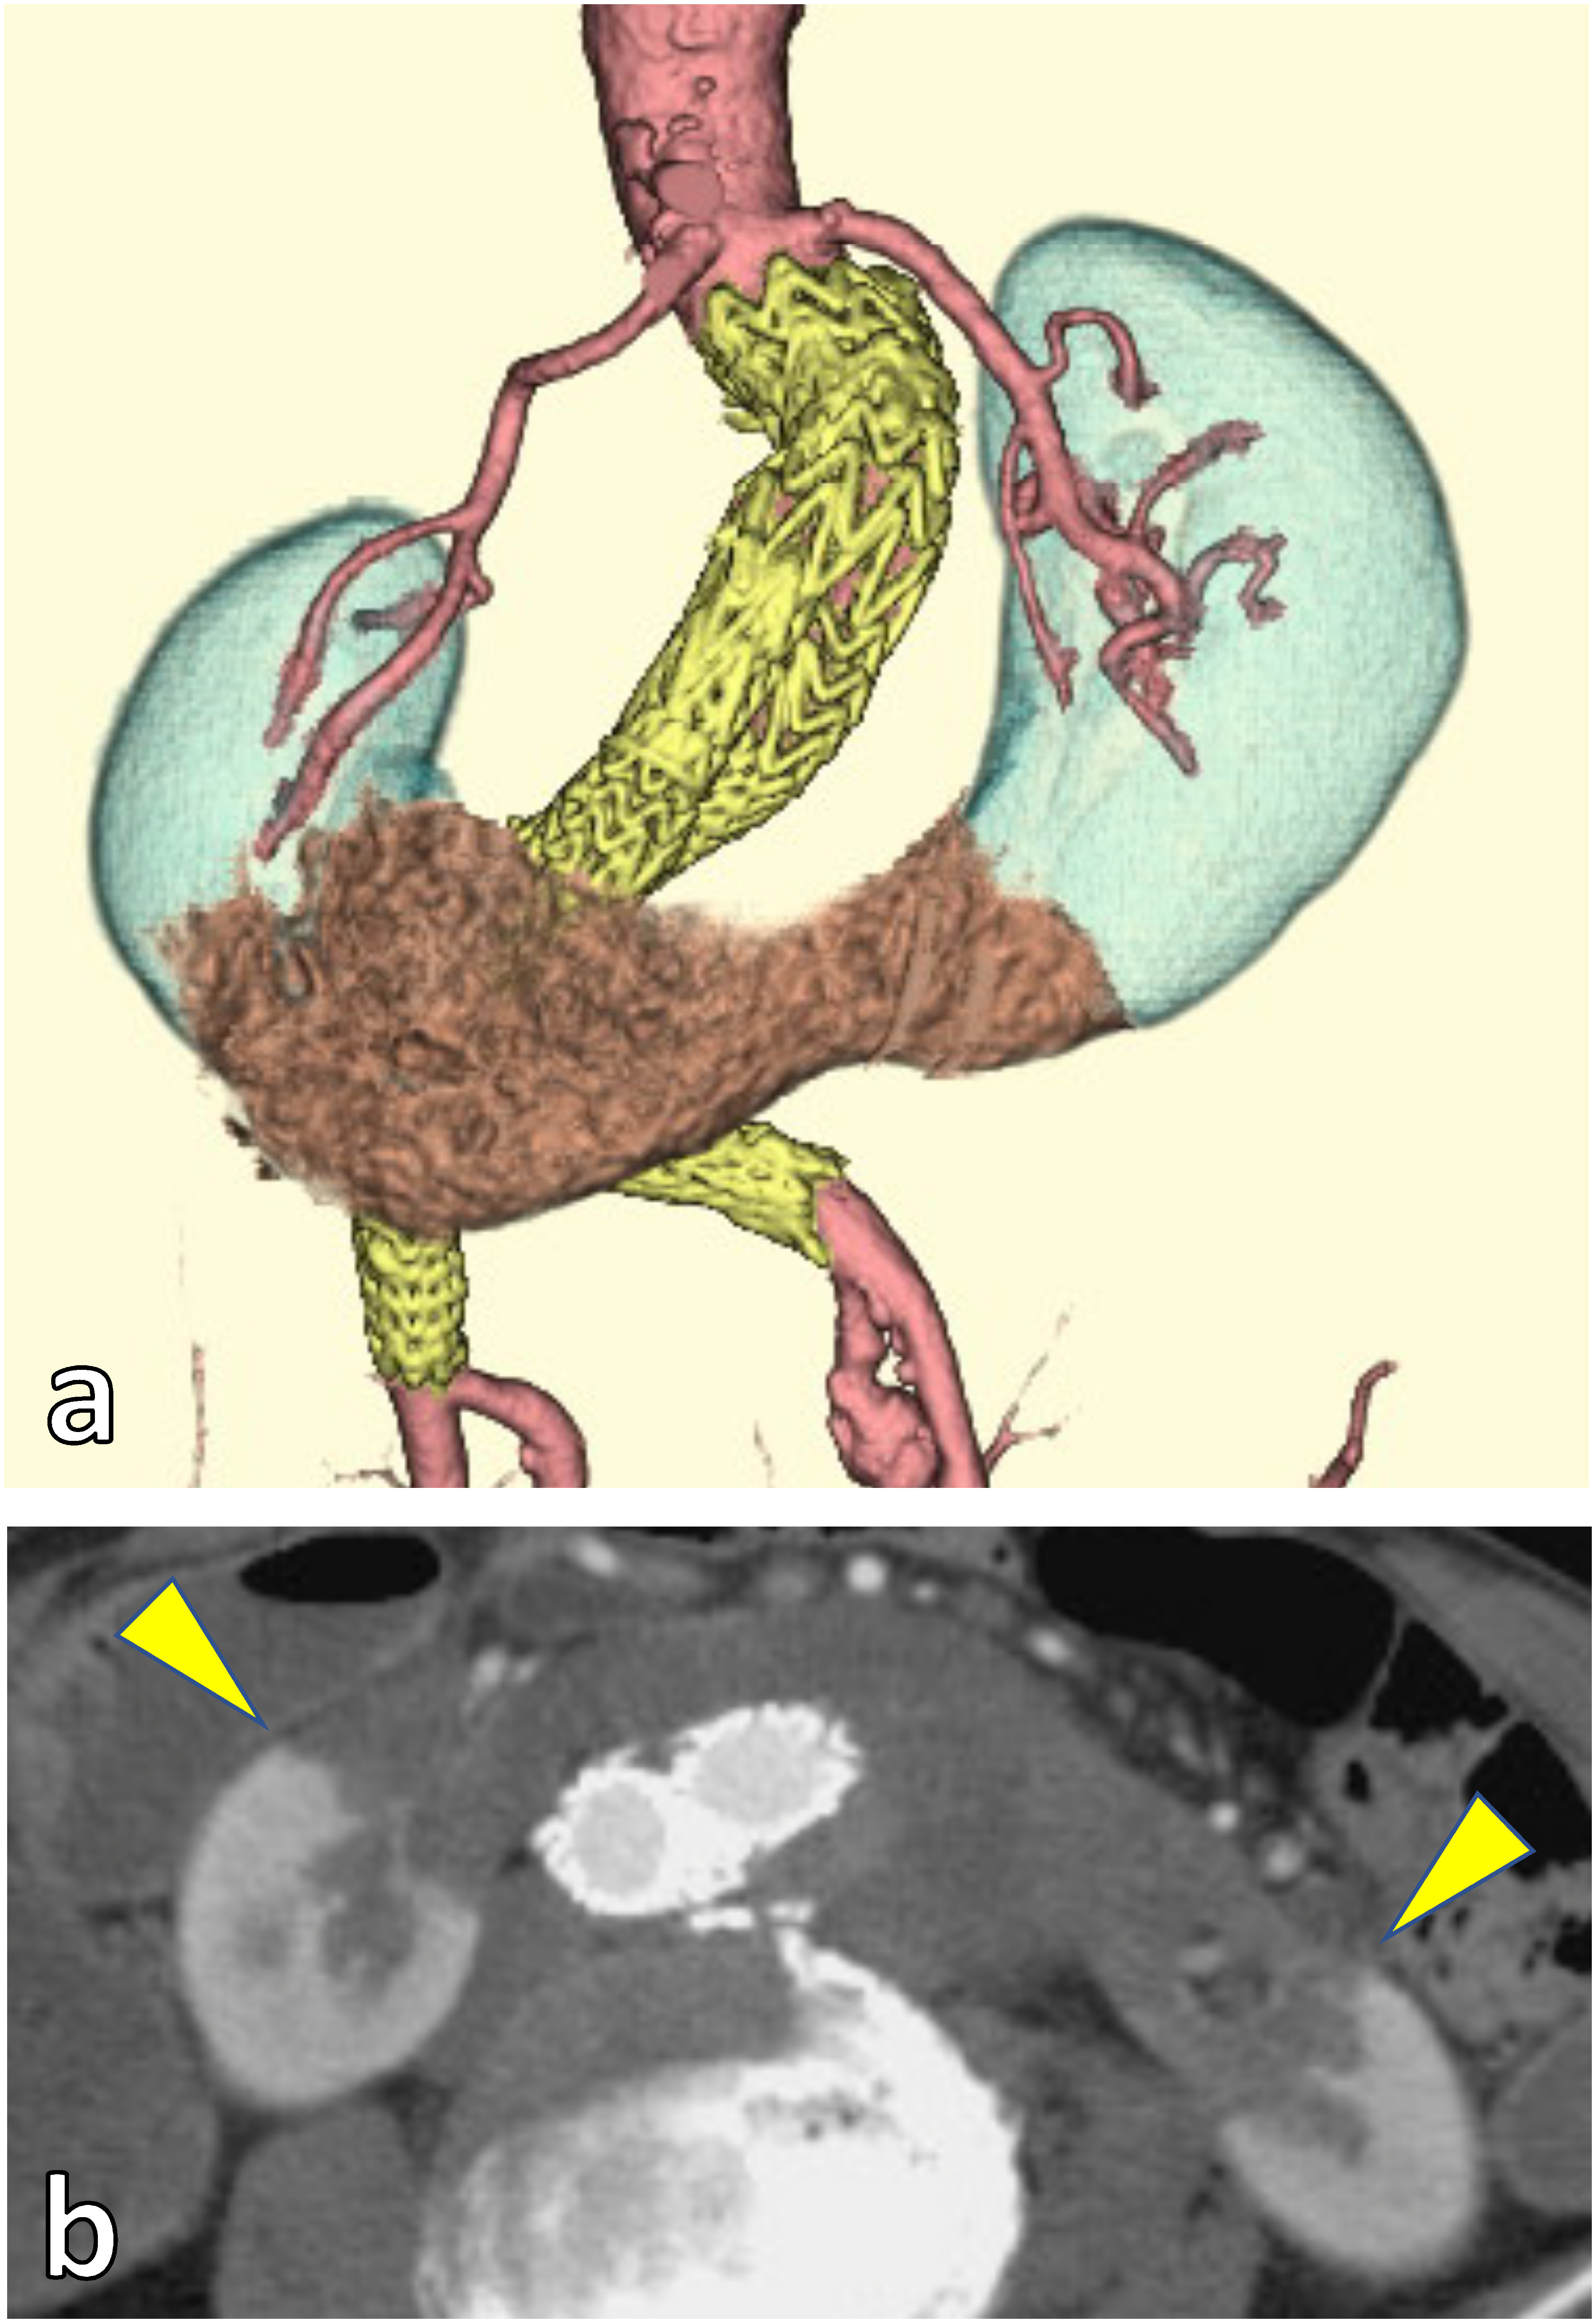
Fig. 2 Postoperative contrast-enhanced computed tomography and ischemia of the isthmus. (a) Postoperative three-dimensional reconstruction shows endovascular abdominal aortic aneurysm repair and ischemia of the isthmus (brown area) by occlusion of accessory renal arteries. (b) Postoperative contrast-enhanced computed tomography shows infarction of the isthmus in the same area between the watershed lines (arrow heads). The estimated infarction volume of the isthmus was 24.2% (84.18 cm^3^) of the total parenchyma (384.87 cm^3^).
